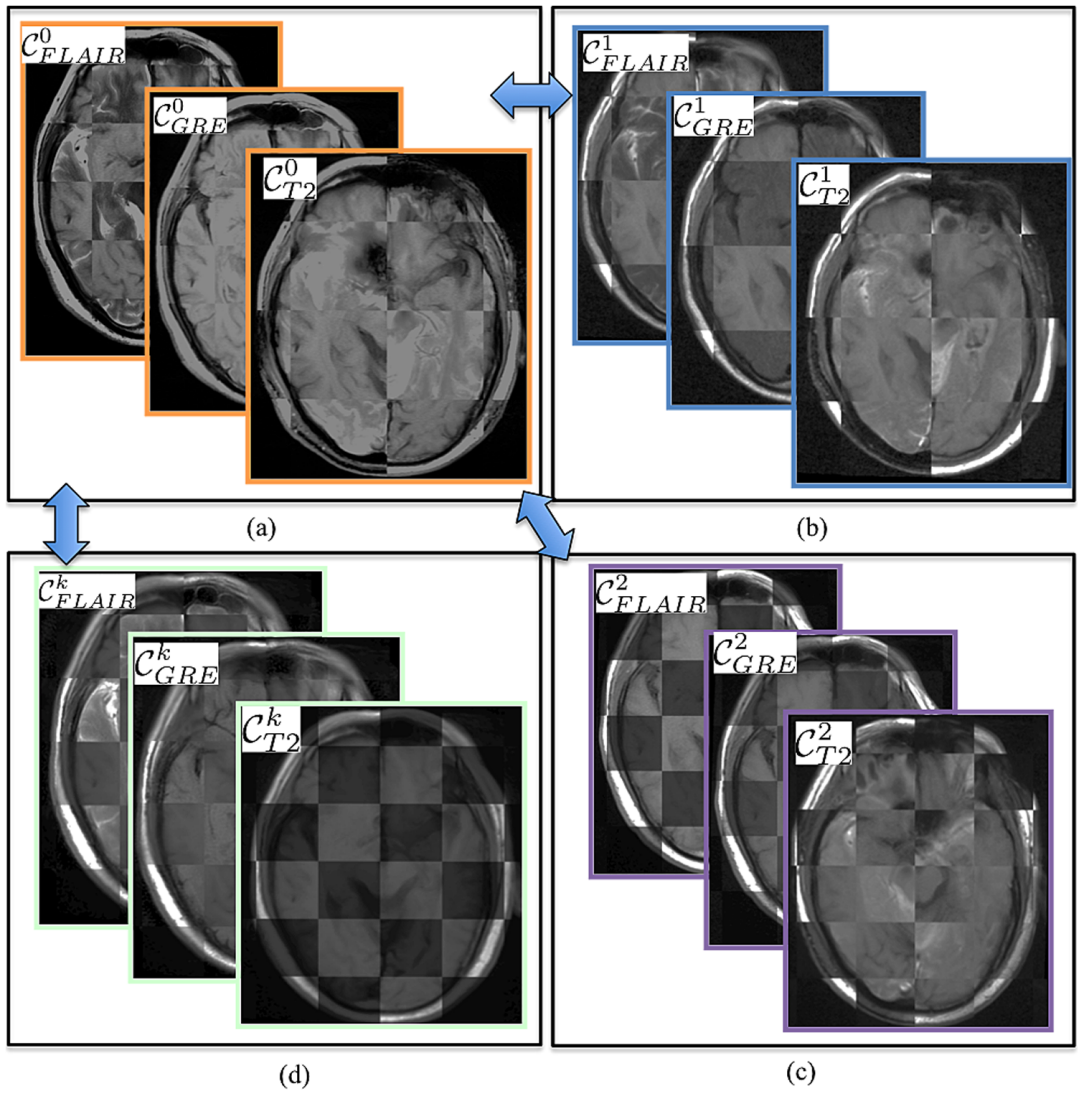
Checker-box representation of co-registration across different MR sequences at different time-points with respect to pre-LITT T1w MRI for (a) different pre-LITT MRI acquisitions, (b) 24-hour post-LITT MRI acquisitions, (c) 1-month post-LITT MRI acquisitions, and (d) 3-month post-LITT MRI acquisitions. Note the perfect alignment of the boundaries across different protocols and time-points. Pair-wise co-registration with respect to one reference MR sequence (T1w MRI) allows for quantitative comparison of different protocols at different time-points.
